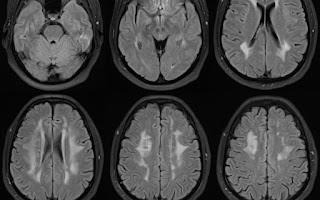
MRI of the brain showing large periventricular confluent white matter high signal intensity on FLAIR sequence
